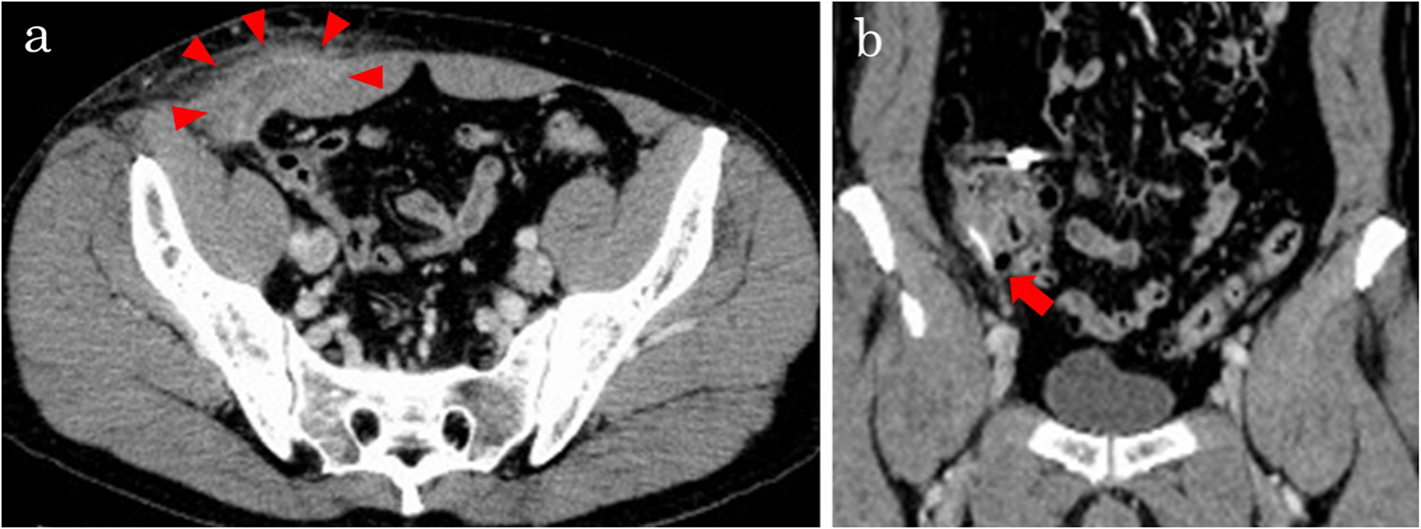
Two months after the initial treatment. a Computed tomography scan shows the same low-density area in the same location (arrowheads). b The foreign body remains in the same location (arrow)
